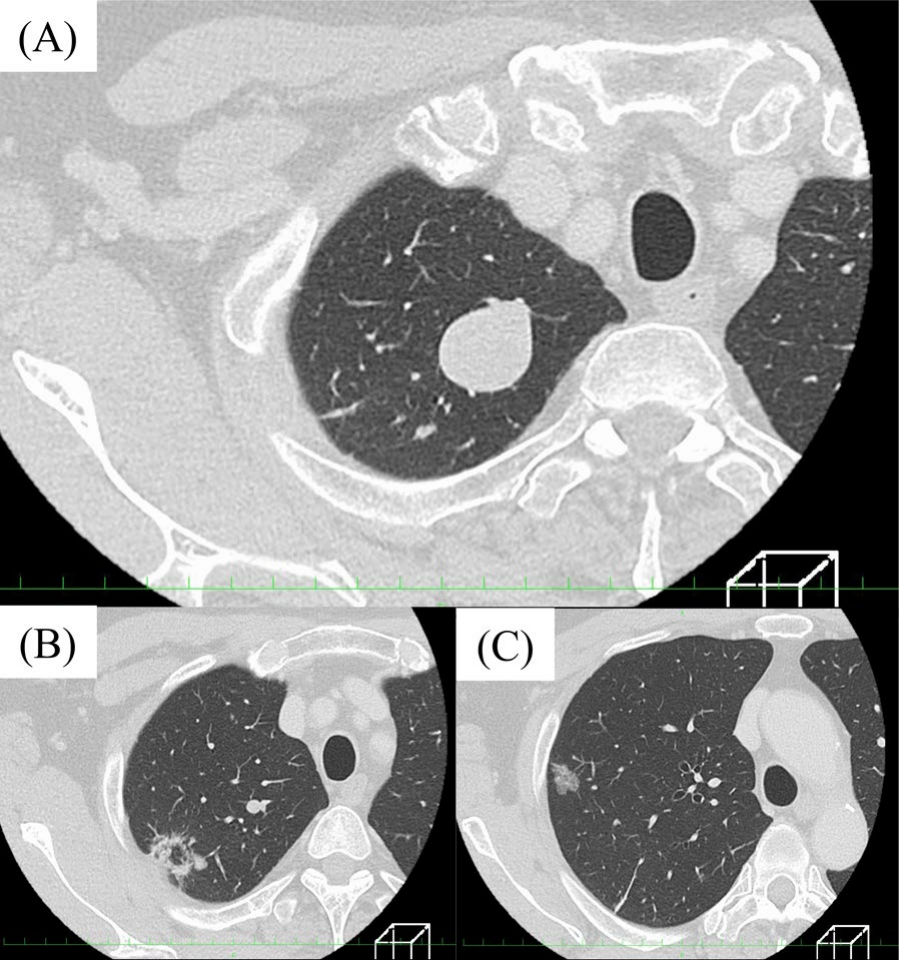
A computed tomographic scan of the chest shows showed a well-defined nodule with punctate calcification (A) and two ground-grass nodules with solid component (B)
